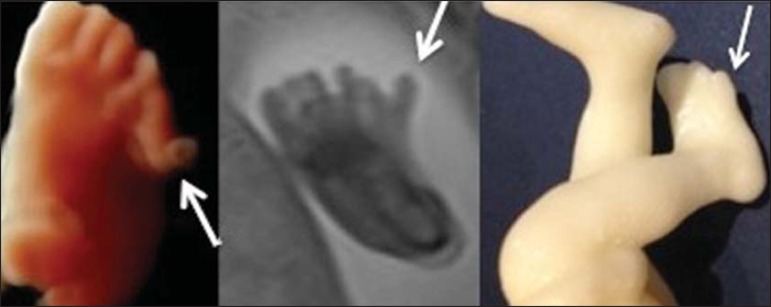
Polydactyly of the foot identified by 3D-US, by MRI, and in the physical
